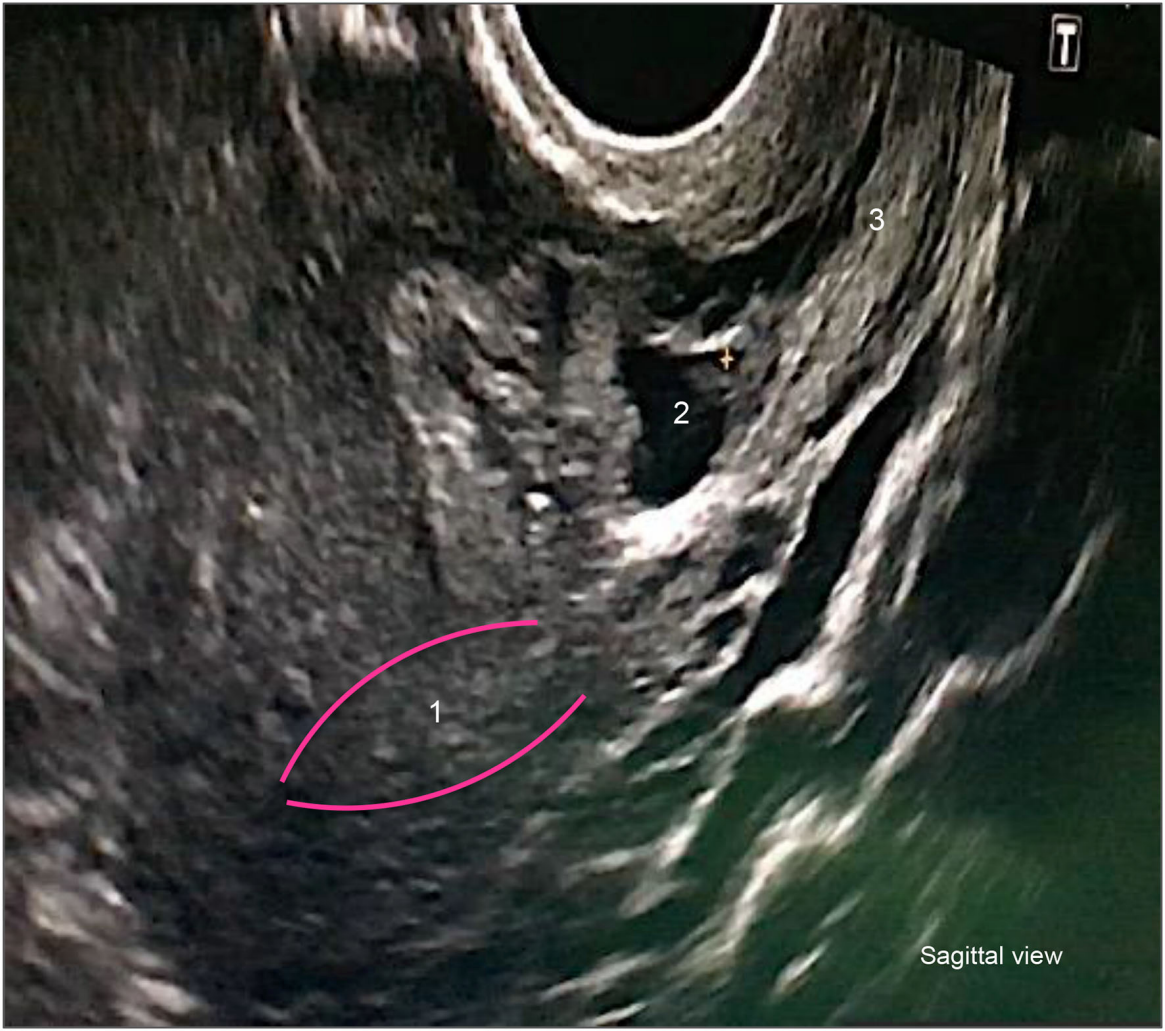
Cesarean scar pregnancy—Sonography. 1. Uterine cavity. 2. Cesarean scar pregnancy. 3.Cervix.
